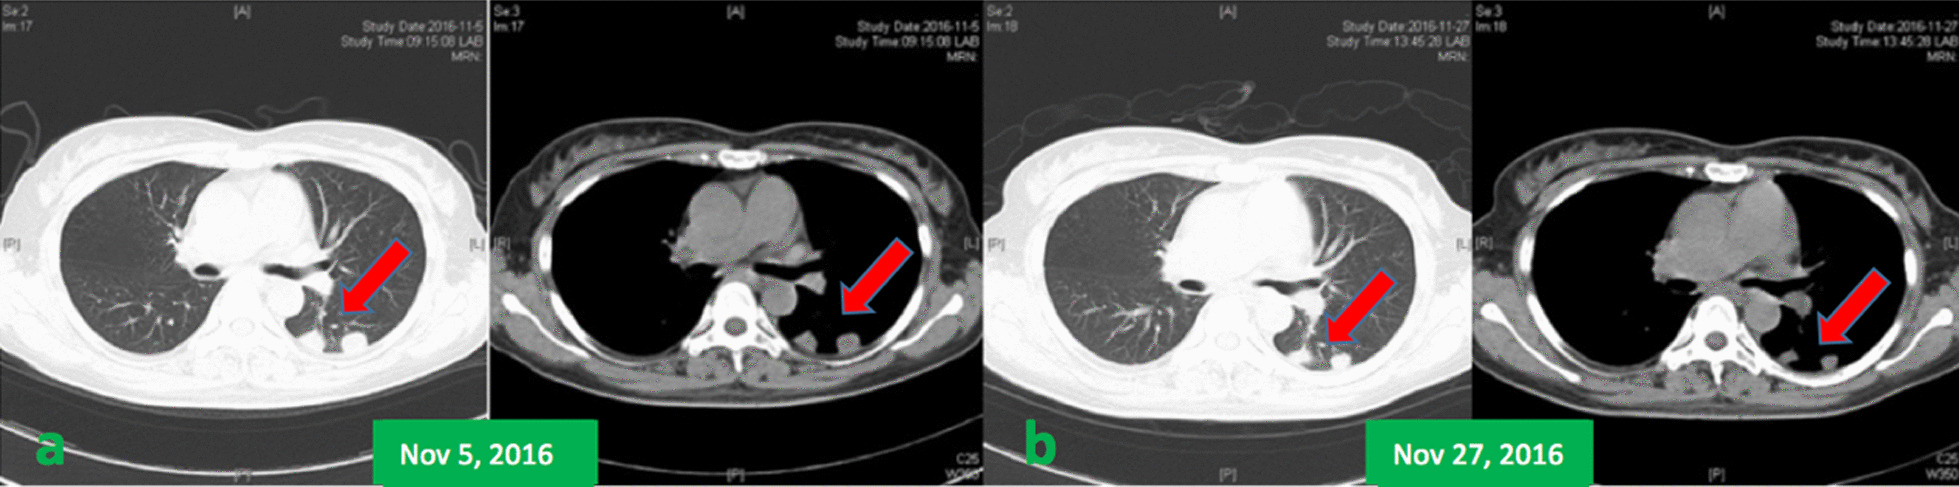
a CT scan showing multiple nodules in the left lung. b CT scan showing a reduction in lung lesions. Red arrows point to the lesions
